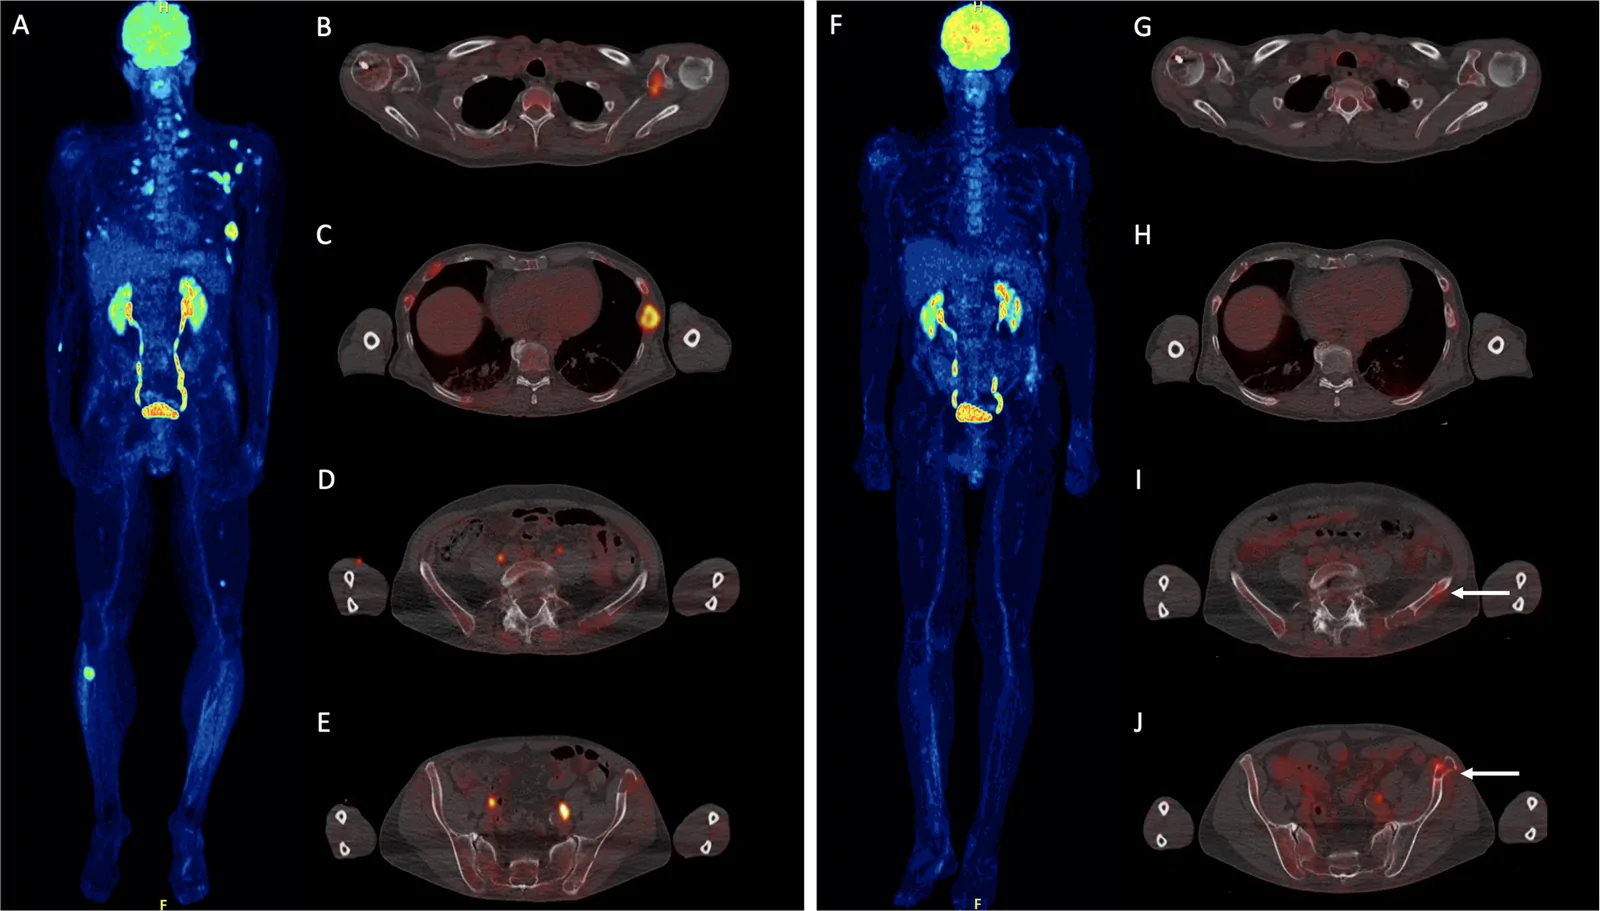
[^18^F]FDG PET/CT of a 66-year old male patient with MM, ISS stage II and R-ISS stage II, before (A-E) and after (F-J) induction treatment with lenalidomide, bortezomib, and dexamethasone (RVd). At baseline, PET/CT demonstrated multiple intensely hypermetabolic focal myeloma lesions, including involvement of the left scapula (B) and ribs, partly associated with soft tissue extension consistent with paramedullary disease (PMD) (C). In addition, lytic lesions with mild [^18^F]FDG uptake were observed, for example in the left iliac bone (D, E). Following induction therapy, the patient achieved a partial response according to IMWG criteria, and achieved MRD negativity. All focal myeloma manifestations showed complete metabolic remission according to IMPeTUs criteria (G, H). Concurrently, two new [^18^F]FDG-avid fractures developed in the left iliac bone (I), partly at the site of pre-existing osteolysis (J) (arrows). At the time of analysis, the patient had not shown disease progression and had achieved a PFS of 36.0 months
